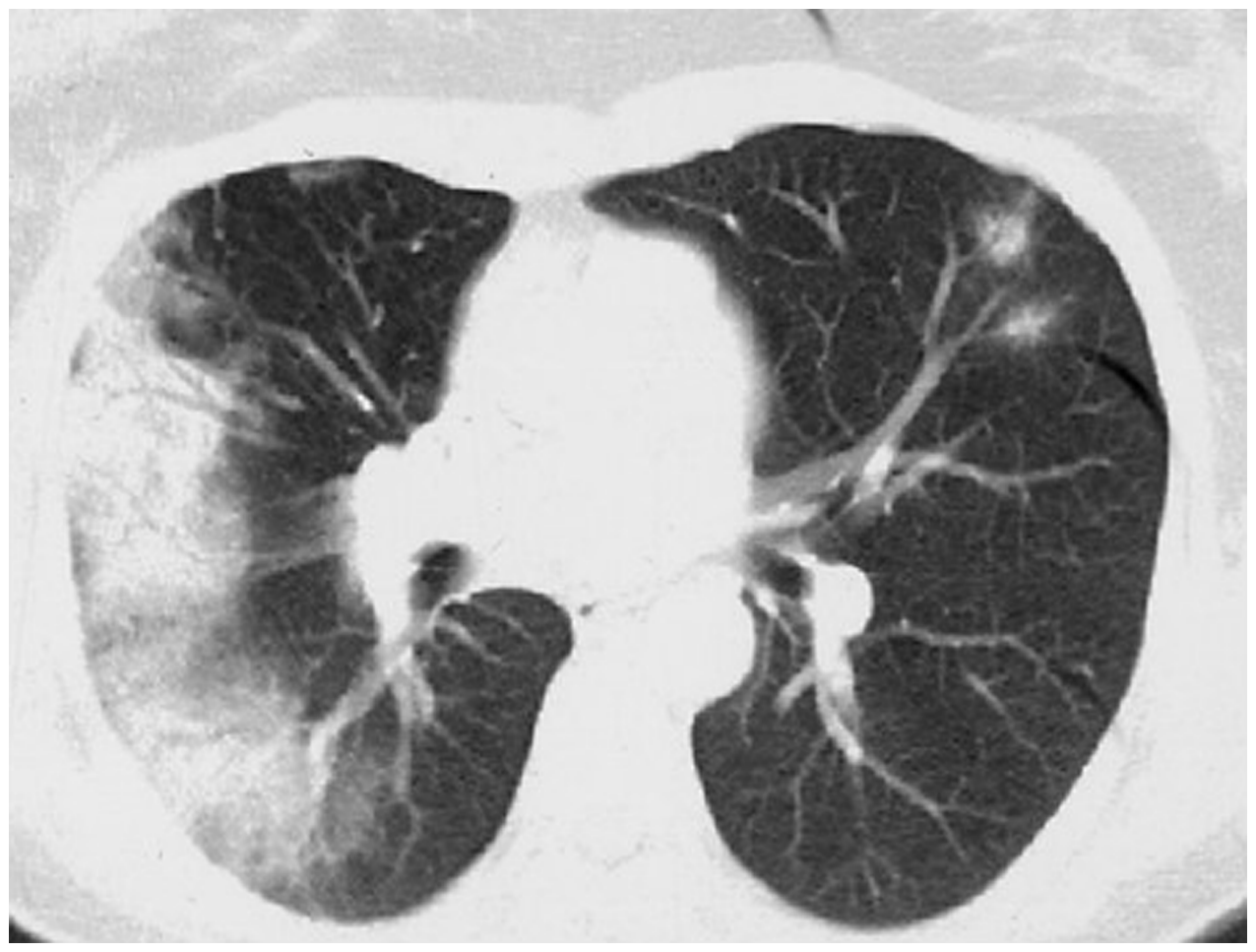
HRCT findings in cryptogenic organizing pneumonia. HRCT pattern shows areas of consolidation and ground glass with some sparing of the immediate subpleural region. This pattern is non-specific and has a wide differential including infection. Migratory (flitting) consolidation in different areas of the lung over days and weeks is a characteristic feature of COP.
